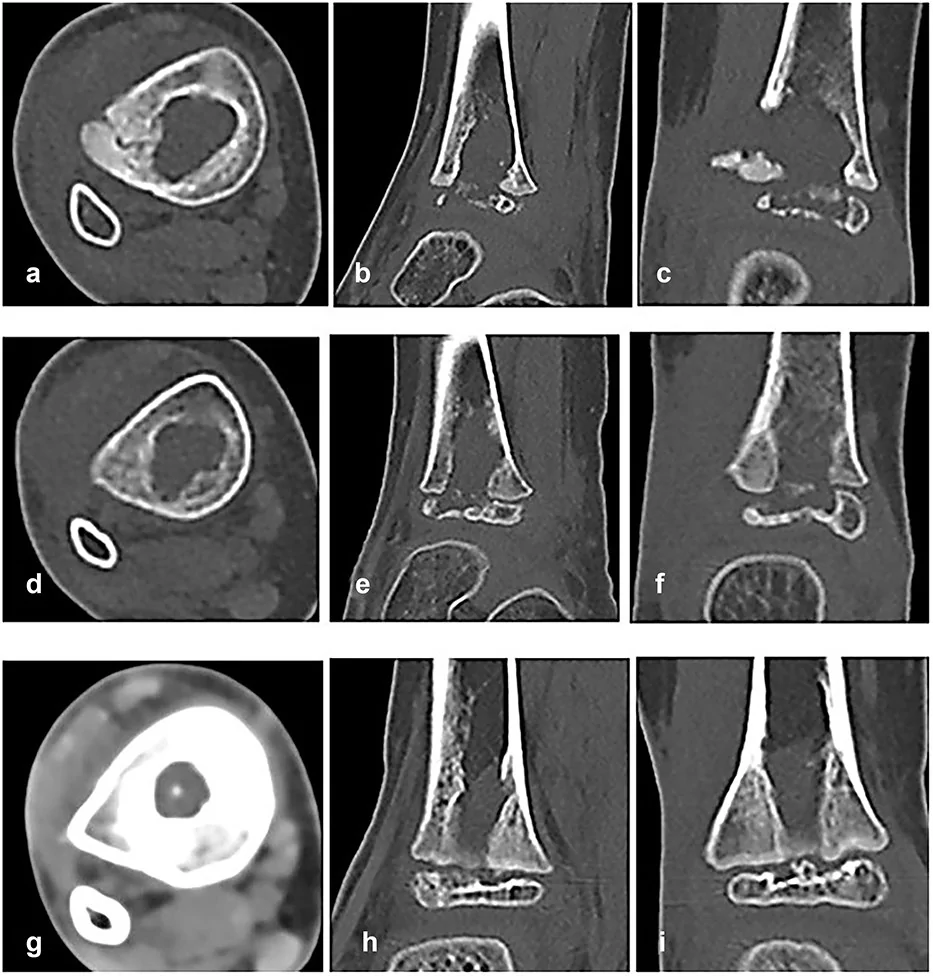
Dynamic CT evaluation in axial, sagittal, and coronal planes through the widest diameter of the lesion; (a–c) pretreatment CT scans: osteolytic metaphyseal lesion with cortical destruction along the lateral contour of the distal tibia; the lesion has well-defined margins with a focally mild osteosclerotic rim formation; maximum dimensions in the three planes are 18 × 15 mm, with a partially destroyed physis and an additional epiphyseal focus measuring 5 × 10 mm; the lesions are filled with homogeneous, structureless material of soft-tissue (parenchymal) density; (d–f) CT scans obtained 6 months after surgery showing a postoperative defect in the metaphysis and epiphysis with well-defined margins; the epiphysis appears fragmented and markedly comminuted; (g–i) CT scans at 18 months after surgery persistence of the postoperative metaphyseal defect with significantly reduced dimensions; the previously fragmented epiphyseal portions have consolidated into a unified structure; the physis is clearly delineated, with no evidence of secondary epiphysiodesis.
